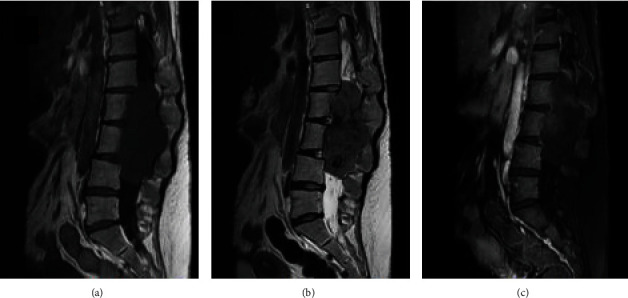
A lumbar spine MRI sagittal ((a) T1, (b) T2, and (c) T1 with contrast).
